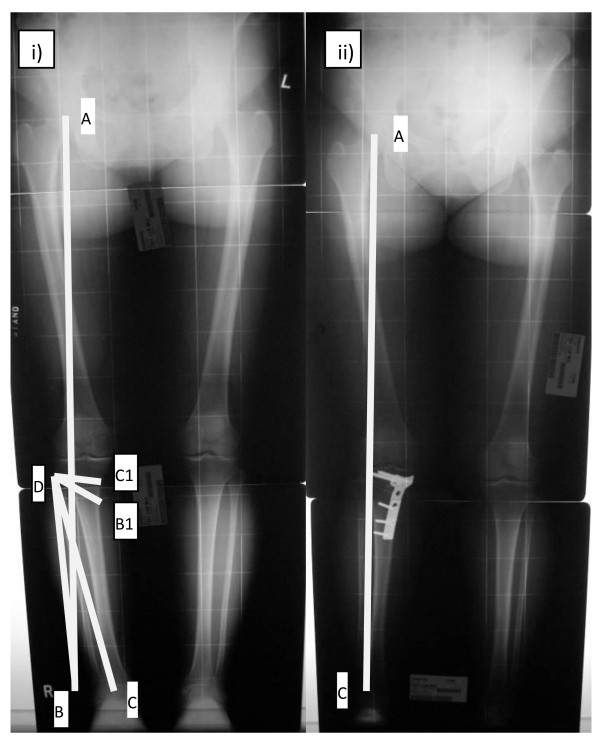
i) Preoperative planning. A: femoral head center; AB: Fujisawa line; C: ankle center; D: Pivot point. Tibial shaft tracing distal to DC1 rotated laterally until point C lying on line AB, DC1 intersected medial tibial cortex to form point B1. C1B1 was the size of posteromedial bone graft. ii) Postoperative radiograph. Line AC intersected the knee joint at 30% lateral to knee center.
